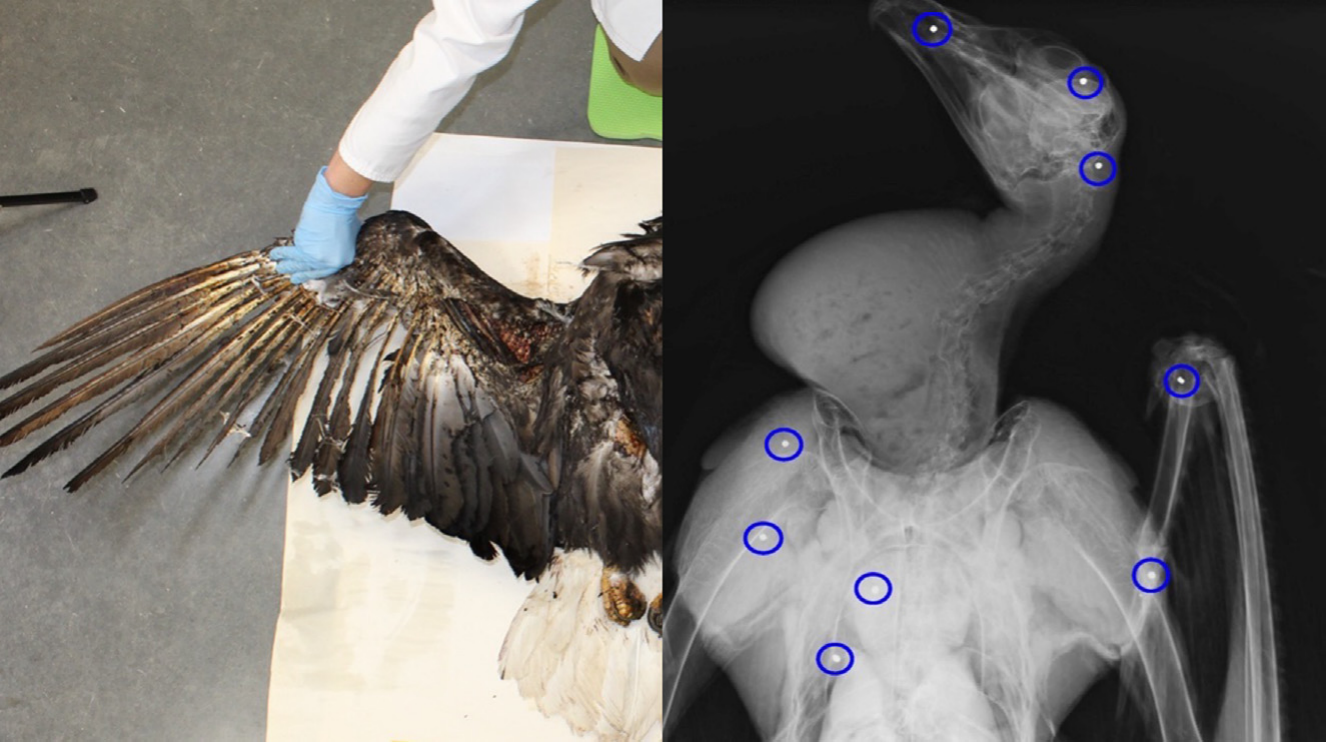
Photograph and radiograph of a bald eagle found dead along a power line near Jordan Valley, Oregon Because of the extensive singeing of the feathers on the wings and body (see photograph), the bird was initially assumed to have died from electrocution. Radiographs of the carcass revealed numerous pellets (in blue circles) in association with fresh wounds, suggesting the bird died from gunshot and then contacted the power lines as it fell to the ground.
